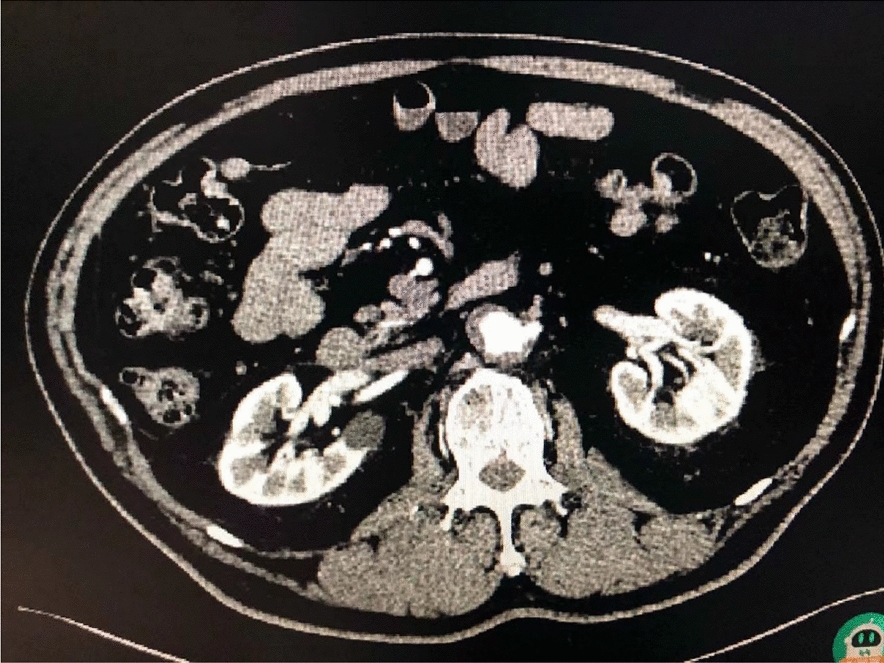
Postoperative abdominal CT shows no sign of local recurrence or distant metastases
